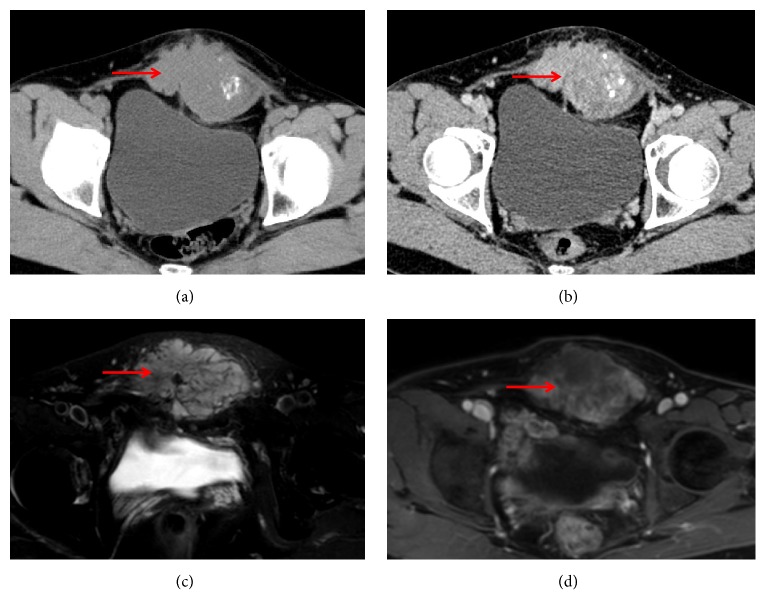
A 44-year-old woman with extraskeletal myxoid chondrosarcoma of the lower abdomen (red arrow). Transverse CT scan showed low density, irregular mass with spot-like calcification (a). Axial contrast-enhanced CT shows that this mass is with moderate enhancement (b). The lesion exhibited hypersignal intensity on T2-weighted sequences (c) and heterogeneous enhancement after injection of gadolinium (d).
